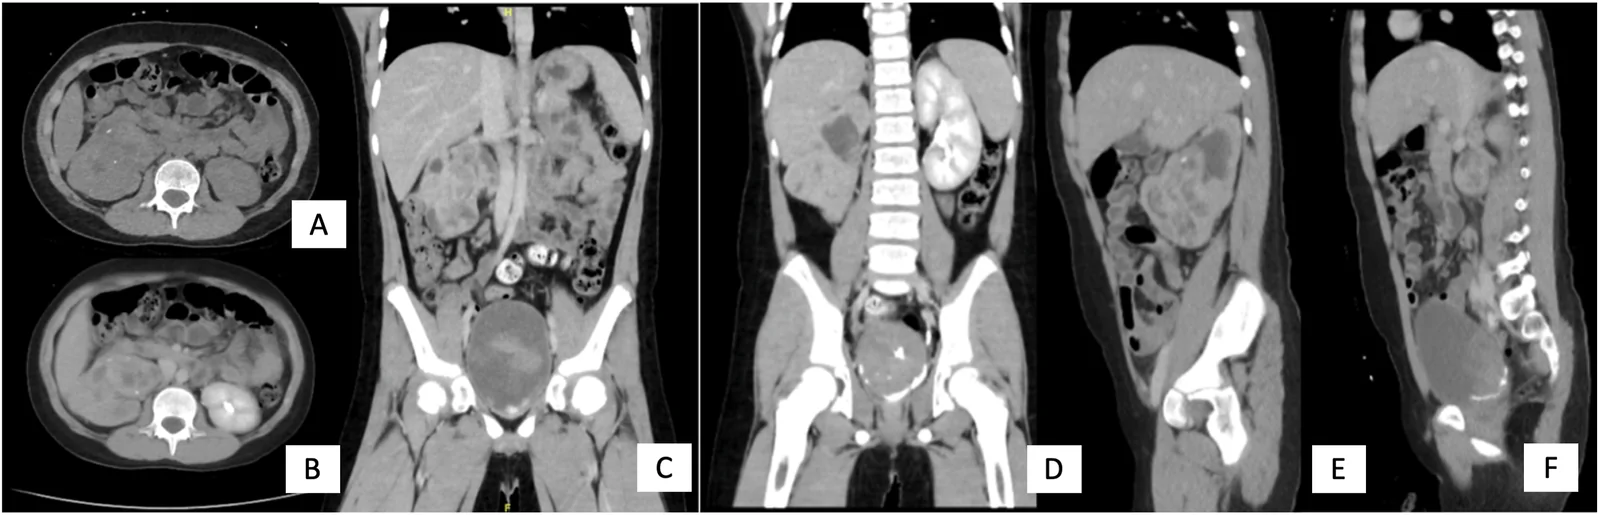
Non-contrast abdominal CT scan. Pre-contrast transverse section (A), post-contrast transverse section, coronal section (C-D), sagittal section (E-F). This finding showed mass on the right kidney with suspected of Wilms tumor.
